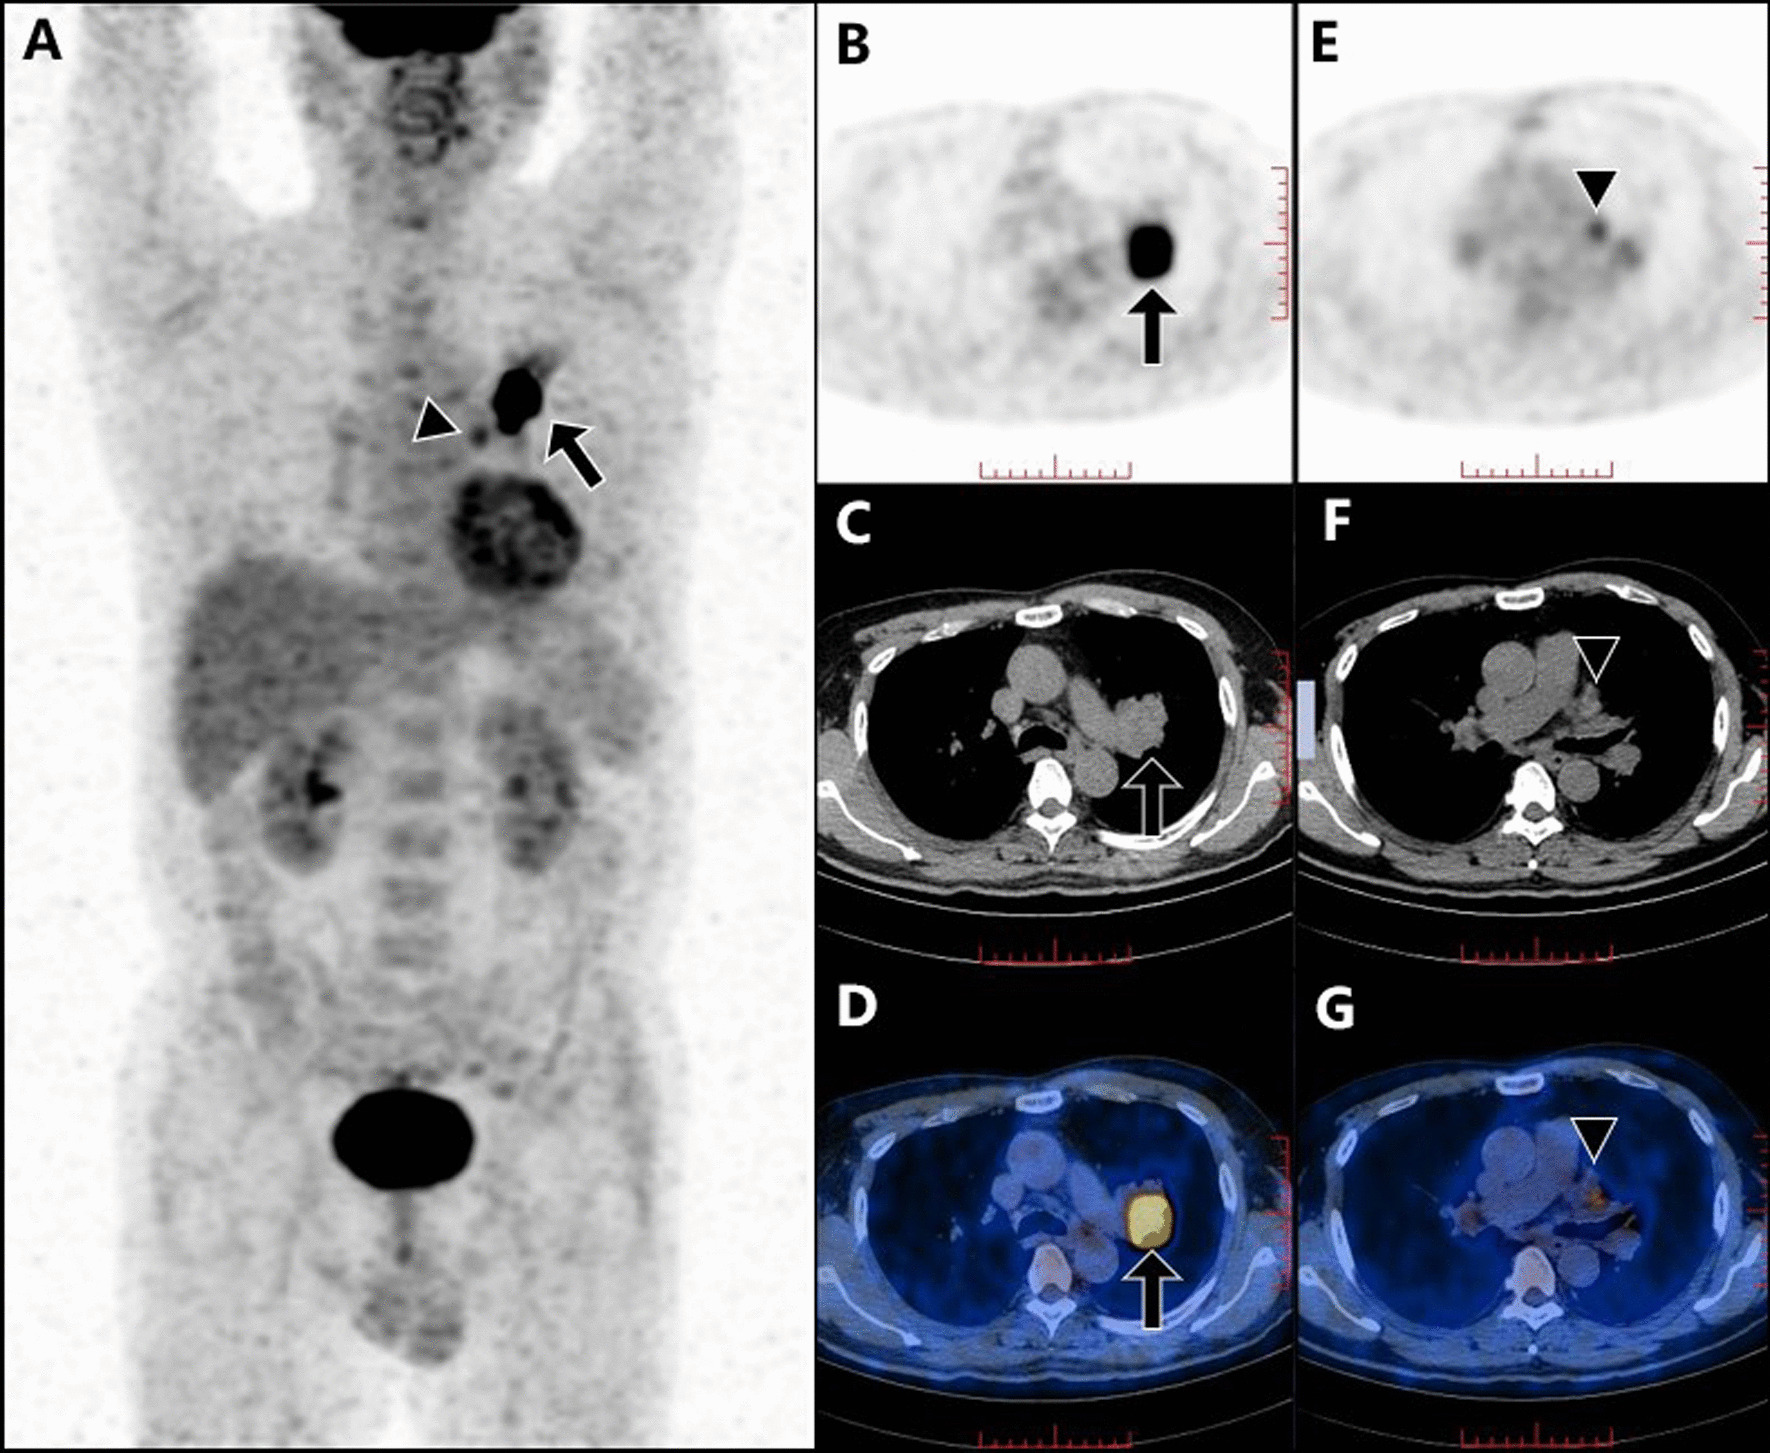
An elderly male (A–G) with high-level SUVmax (3.20) of mediastinal lymph node, high-level primary-tumor SUVpeak (9.48), high serum CEA (5.25 ng/ml) and low serum SCC (0.70 ng/ml). The primary tumor, which was confirmed as moderately differentiated squamous cell carcinoma of the lung, is shown in ^18^F-FDG PET/CT maximum intensity projection (MIP) (A), PET (B), CT (C) and PET/CT (D) images (arrows). The mediastinal lymph node in area 5, which was the highest SUVmax (3.20) node in the mediastinum and proved to be metastatic by postoperative pathological analysis, was displayed in ^18^F-FDG MIP (A), PET (E), CT (F) and PET/CT (G) images (triangle)
